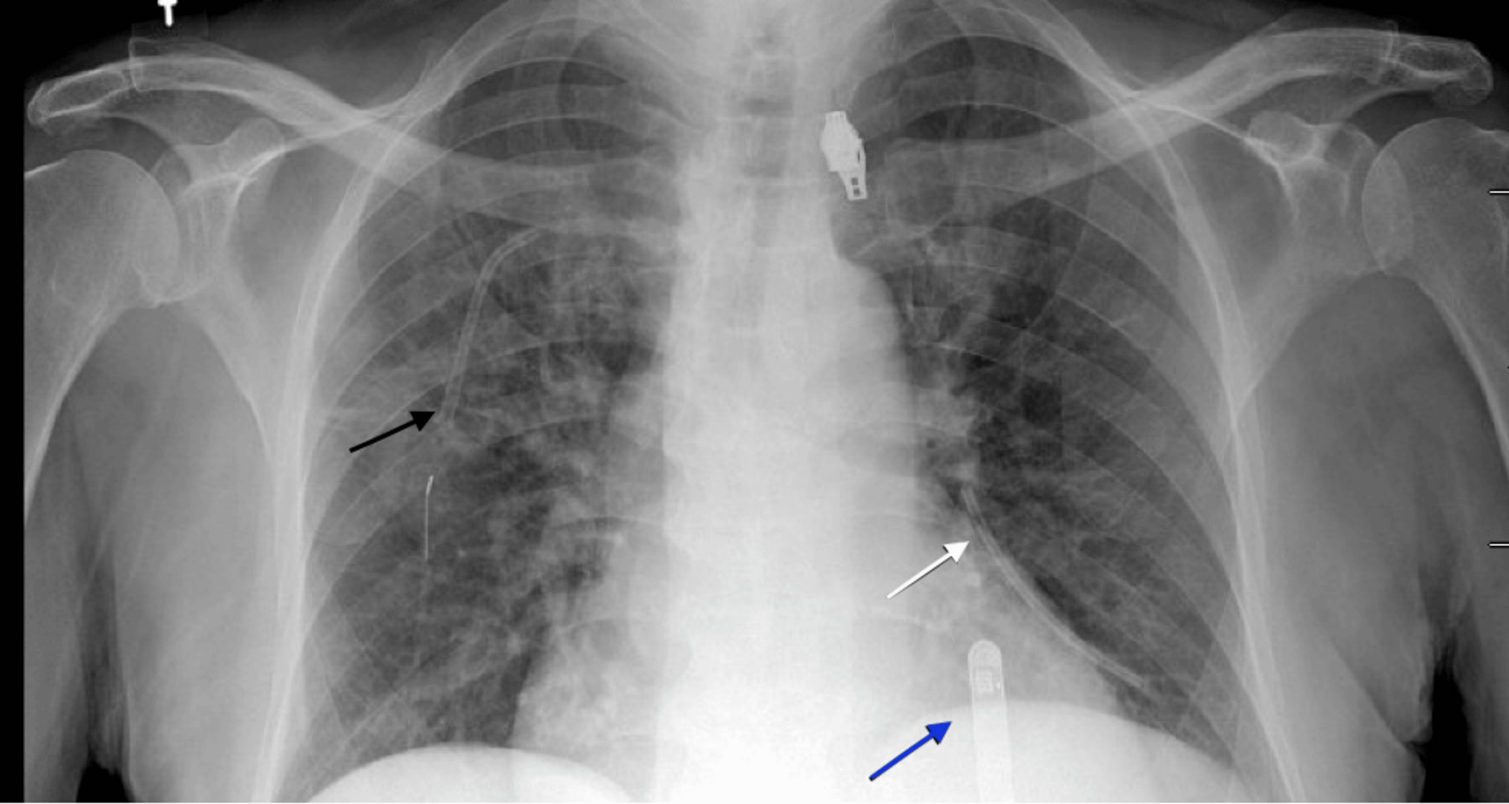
Preoperative chest X-ray Demonstrating the proximal central venous access catheter within the right subclavian vein (marked by a black arrow) and the distal fragmented catheter piece within the left pulmonary artery (marked by a white arrow). Existing loop recorder is in the appropriate position (marked by a blue arrow).
